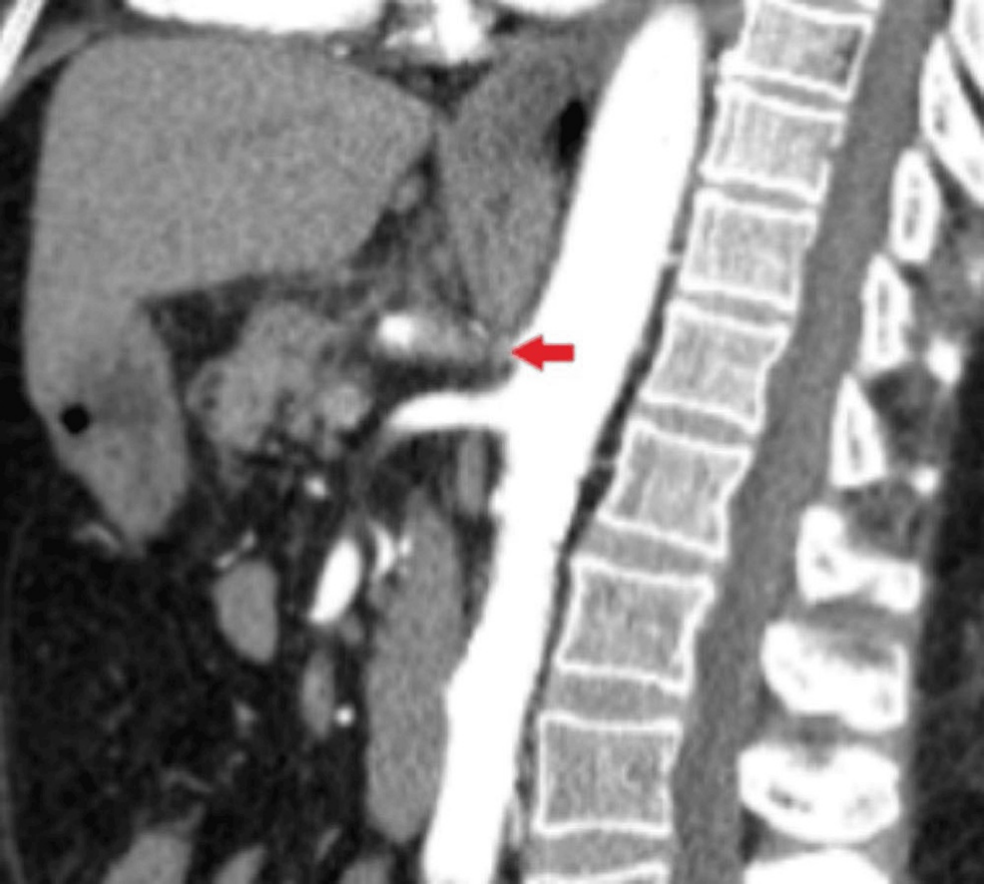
Computed tomography angiography (CTA) of the abdomen showing celiac artery thrombosis.
